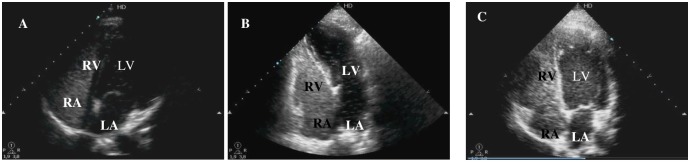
Transthoracic contrast echocardiography and grades of pulmonary shunt. (A) Grade 1. (B) Grade 2. (C) Grade 3. RV, right ventricle; RA, right atrium; LV, left ventricle; LA, left atrium.
